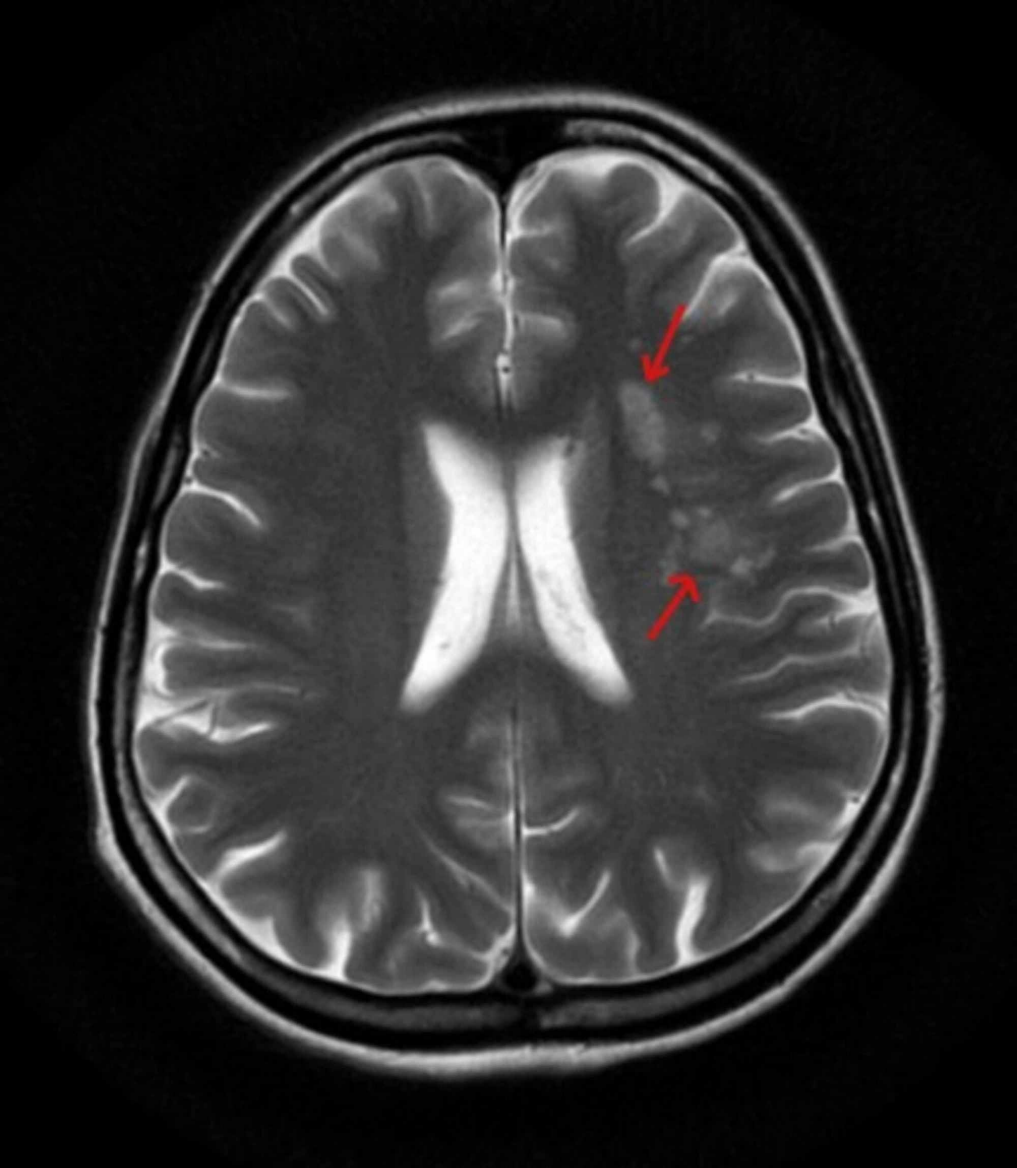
Magnetic resonance imaging (MRI) of the brain showing focal acute infarcts in the left centrum ovale, left corona radiata, and left external capsule in the left middle cerebral artery territory
